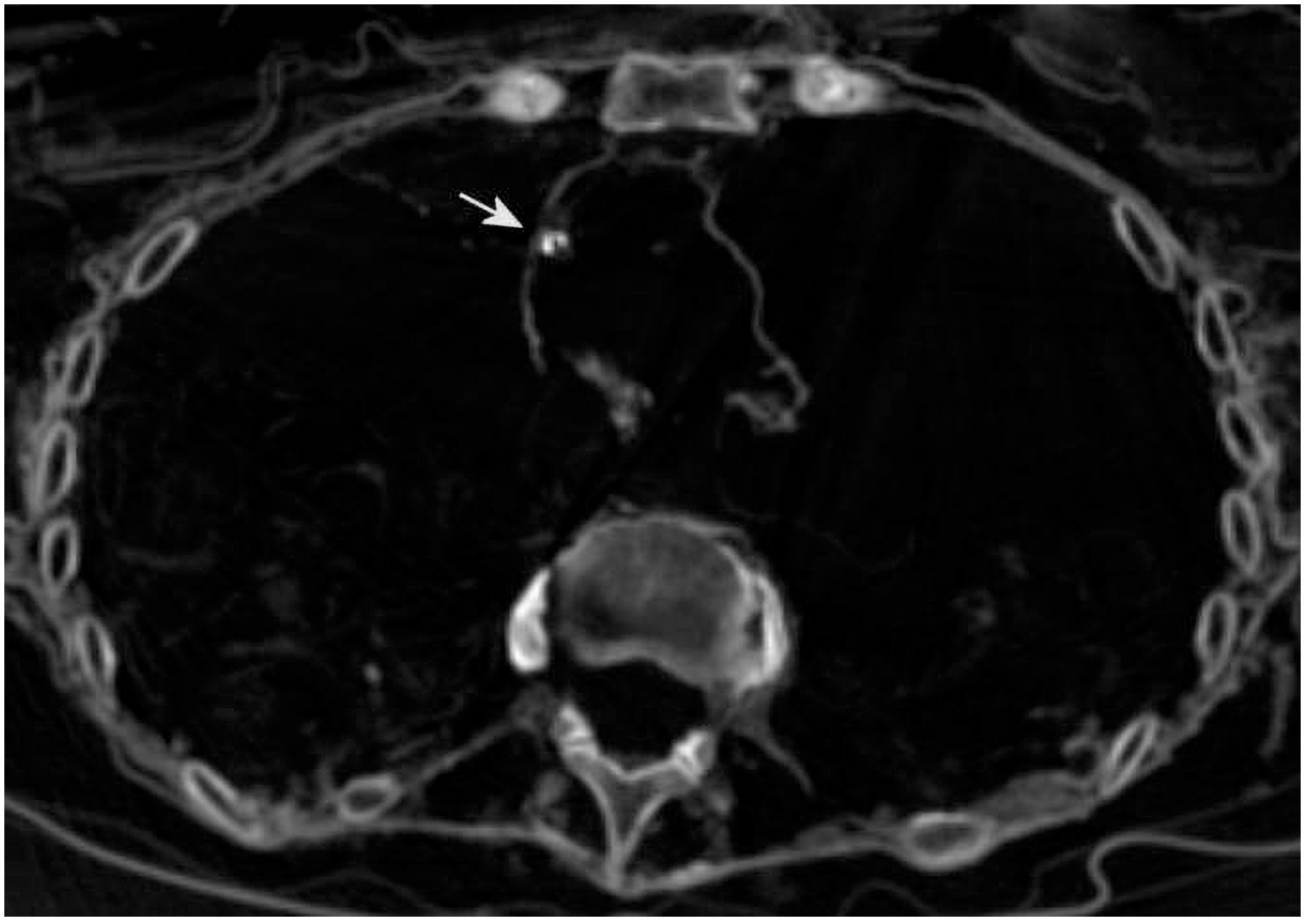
CT scan of the heart showing focal ring-like calcification of one of the coronary vessels (arrow).
